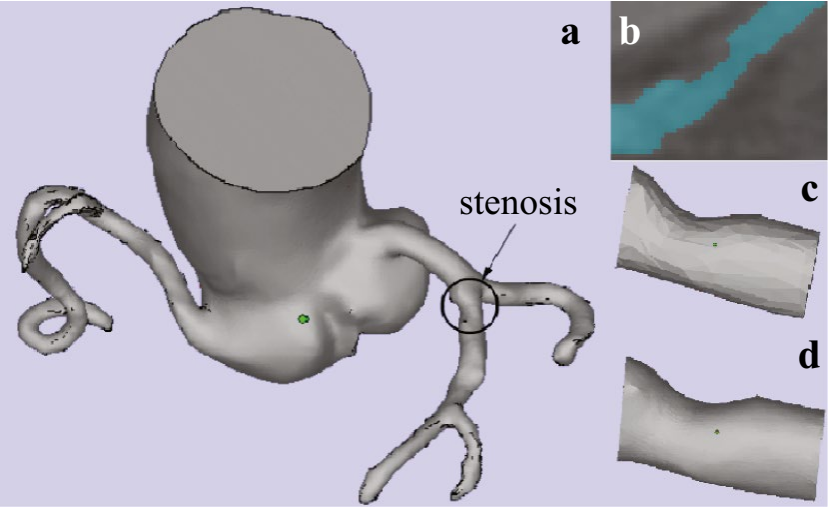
a Coronary vasculature determined from segmentation of CTA images. b Segmentation of an artery section containing that stenosis. (c y d) Representation of the stenosis surface with 1034 (c) 12412 triangles (d). The upper image corresponds to the maximum resolution available when the entire fluid domain is considered. The CTA images were provided by the *Hospital Infanta Cristina* in Badajoz (Spain). They were obtained from a 64 detectors scanner (LightSpeed VCT, General Electric Medical Systems, Milwauke, WI, USA) using the retrospective cardiac reconstruction method (SnapShot Segment Mode) with the following acquisition parameters: slice thickness 0.625 mm, rotation time 0.4 s, tube voltaje 120 kV, collimation 0.625 mm and a pitch of 0.18, 0.20, 0.23 or 0.26 (automatically set by the software depending on the patient’s heart rate)
